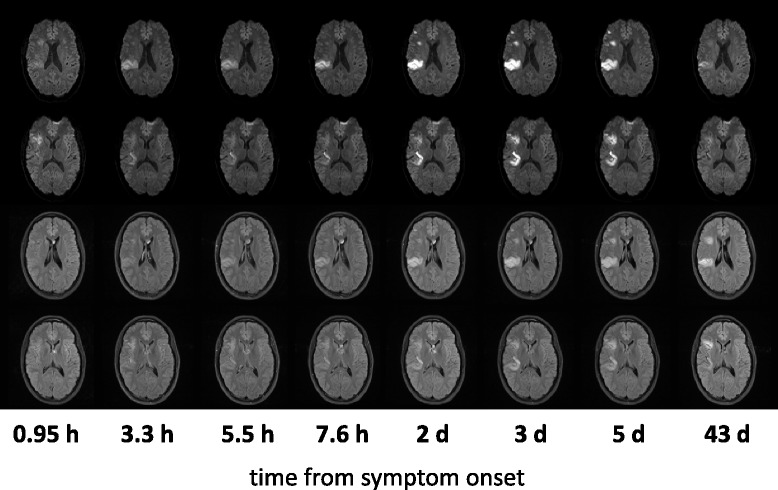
Development of the anterior and the posterior lesion on serial DWI and FLAIR images. The first and second rows show the diffusion-weighted images (DWI) on two slices depicting the lesion development over time from symptom onset. The third and fourth row shows the coregistered fluid-attenuated inversion recovery (FLAIR) images corresponding to the DWI slices
